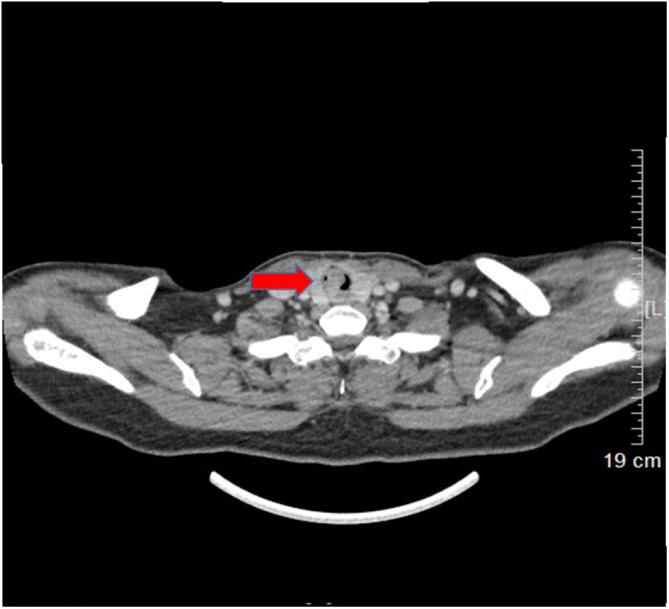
Trachea spiral CT shown a tracheal mass resulting in a 90% obstruction of trachea lumen.
